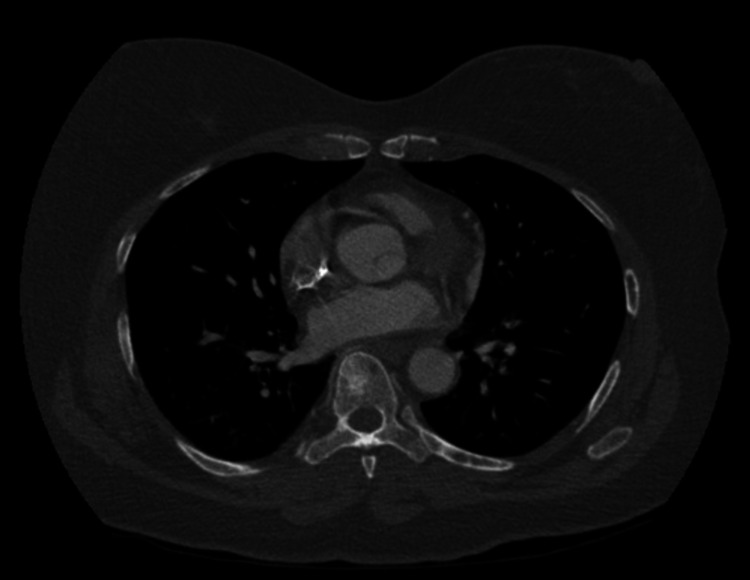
CT angiogram of the chest showing diffuse sclerotic bone lesions consistent with metastatic disease. CT: computed tomography
